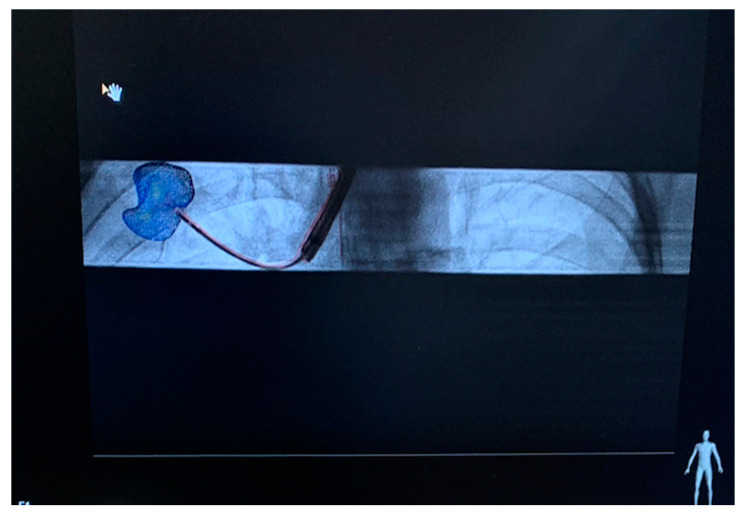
Intraoperative CBCT scan showing the pre-planned tumor location and position.
